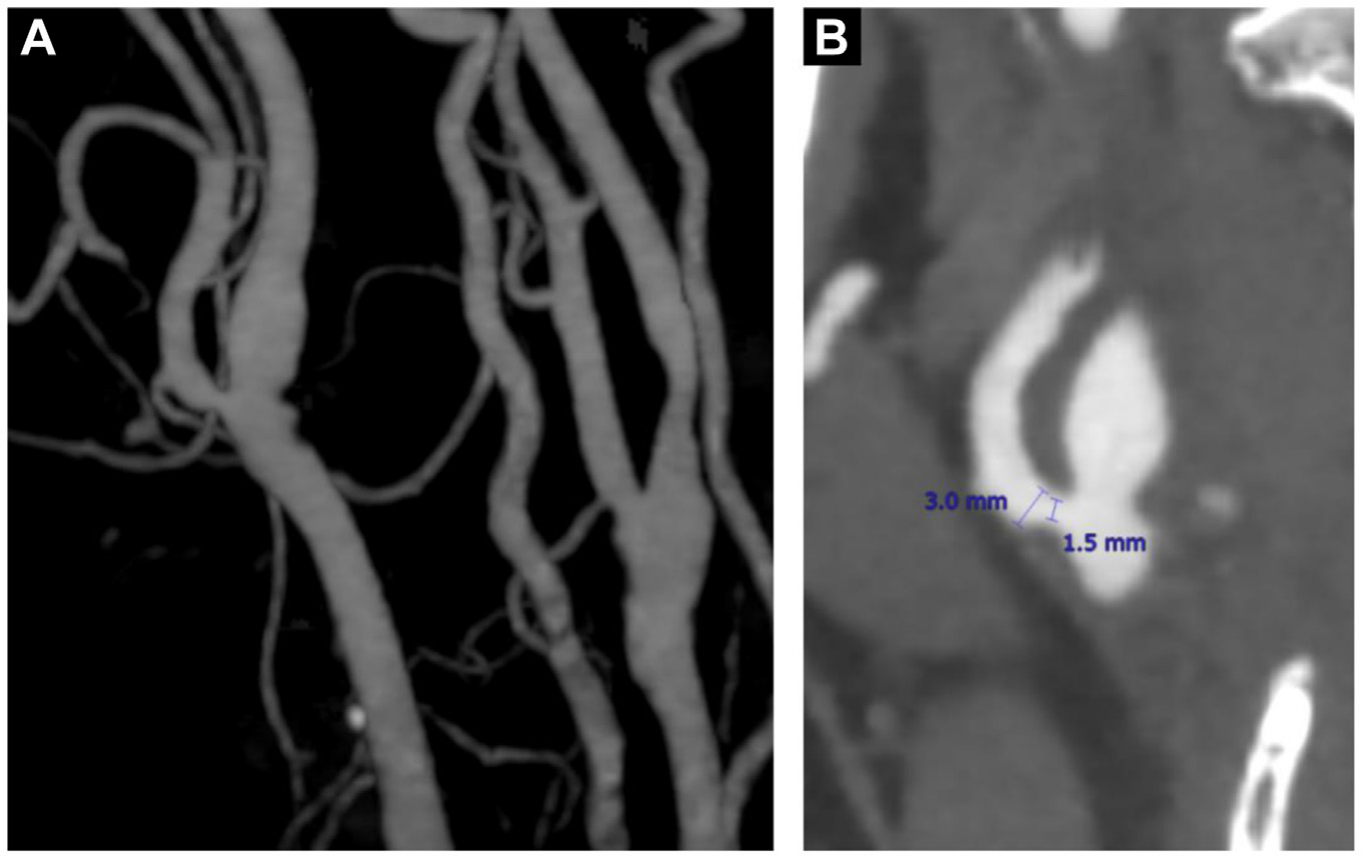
A, Three-dimensional reconstruction of the right external carotid artery (ECA) from computed tomography (CT) angiography before thoracic endovascular aortic repair (TEVAR). B, CT angiography showing right ECA stenosis.
